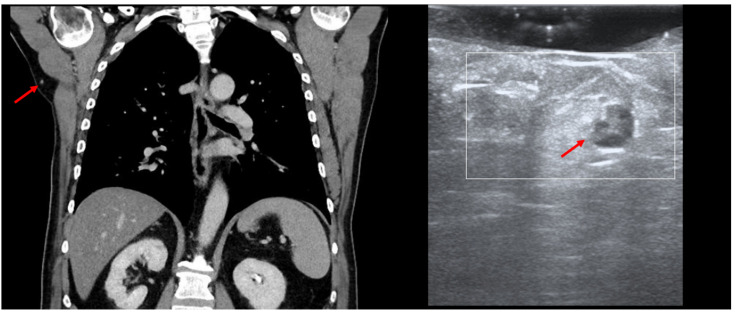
(PET-CT—left, US—right): Thoracic PET-CT showing a new lesion in the right axillary area in a MM patient, suspicious of metastatic disease, as marked by “↑”; Intraoperative HFUS showing a subcutaneous hypoechoic lesion, marked by “↑”, guiding the excision margins—transversal view.
